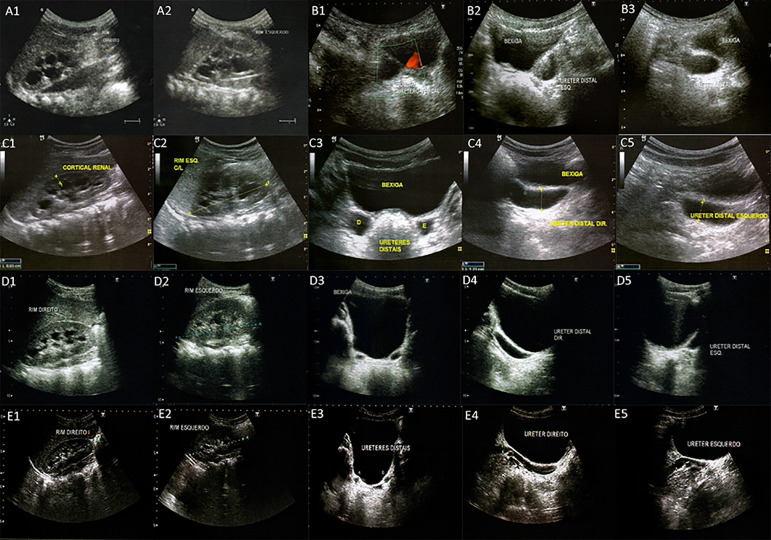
Renal ultrasound showing patient evolution according to serial ultrasonography (US) evaluation. A1, A2. US at 1-month of age showing bilateral hydronephrosis, mainly on the right kidney. Right kidney size: W 50mm x L 29mm x T 20mm. Left kidney size: W 57mm x L 26mm x T 27mm. B1, B2, B3. At 1 year of age, moderate bilateral hydronephrosis with slight reduction of renal cortex thickness and dilation of both ureters entire length, except at the ureteral vesical junction (UVJ), which exhibits a constriction area. Right kidney size: W 65mm x L 34mm x T 33mm. Left kidney size: W 69mm x L 32mm x T 29mm. C1, C2, C3, C4, C5. At 2 years of age, moderate bilateral hydronephrosis, still with slight thinning of renal cortex and dilation of both ureters entire lenght (1 cm on the right side and 0.8 cm on the left), with an abrupt stenosis in the UVJ region of 0.2 cm caliber. Right kidney size: 76mm x 28mm x 28mm. Left kidney size: W 69mm x L 30mm x T 30mm. D1, D2, D3, D4, D5. At 7 years of age, moderate bilateral dilation of both ureters with diameter of 0.8cm and still with important reduction of the distal ureters and UVJ caliber. Mild dilation of the pyelocaliceal system. Renal dimensions within normal values. Right kidney size: W 81mm x L 32mm x T 32mm. Left kidney size: W 82mm x L 34mm x T 33mm. E1, E2, E3, E4, E5. At 12 years, mild pyelocaliceal system ectasia and reduced ureteral dilation. Both ureters with preserved trajectory and with estimated diameter of 0.7cm. Preserved renal cortex.
